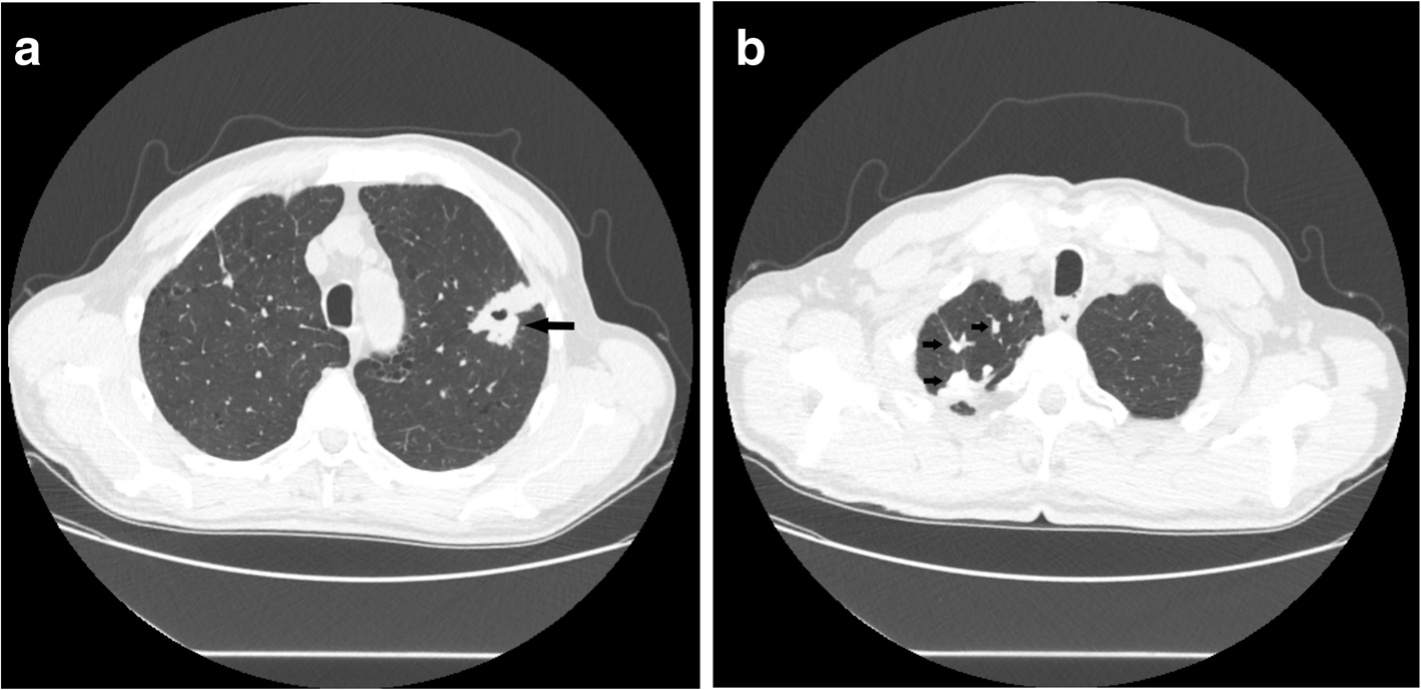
Pulmonary CT. a A cavity lesion (black arrow) in the posterior segment of the upper lobe of the left lung. b Multiple nodules and spots (black arrow) in the upper lobe of the right lung
